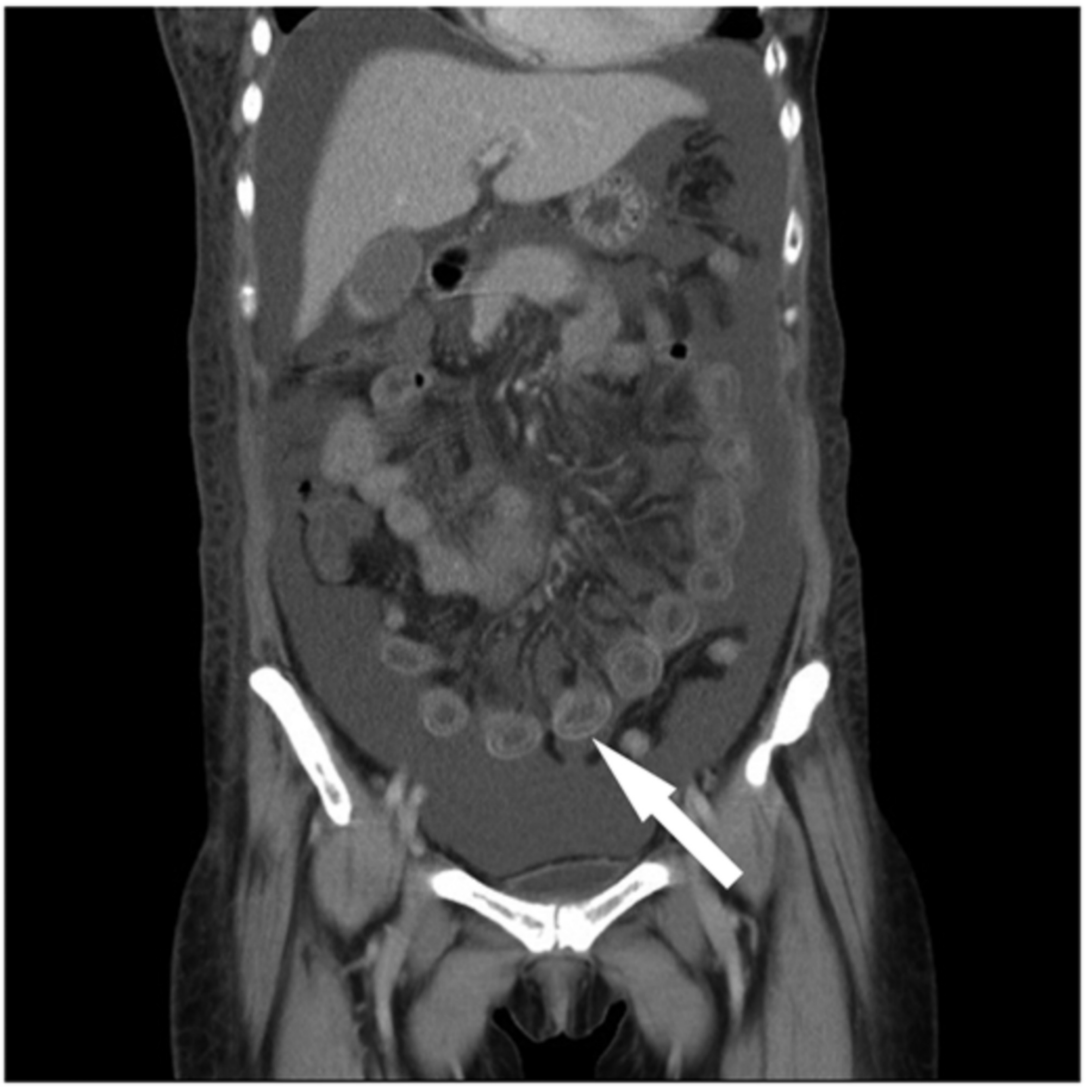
Target sign on CT scan of abdomen/pelvis in lupus enteritis Contrast-enhanced CT of the abdomen (coronal view) revealed target sign, which indicated diffuse circumferential wall thickening with submucosal edema of the entire small bowel, showing the ‘double halo’ or ‘target,’ sign (arrow)
